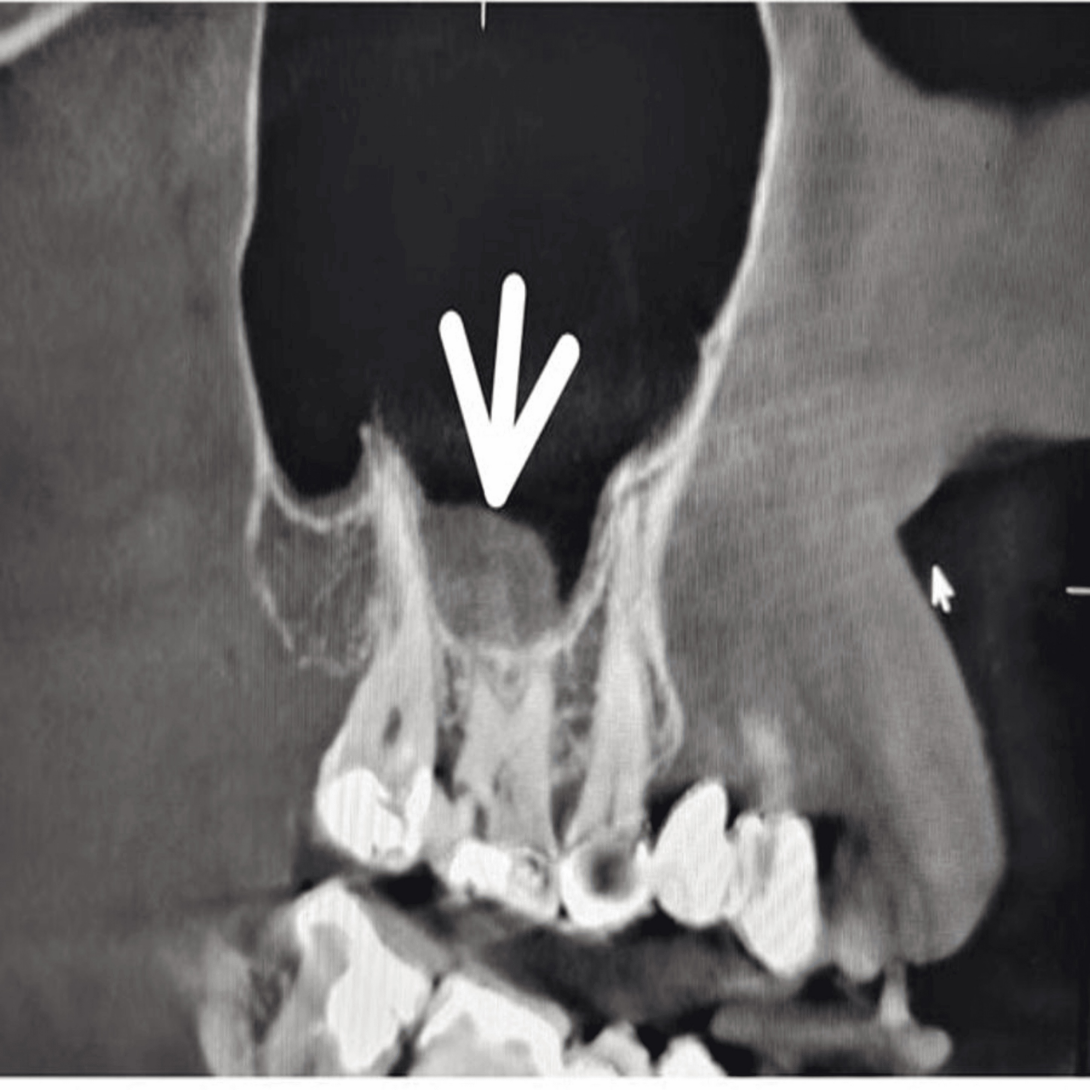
Observation of MSMT in the right MS in CBCT image. MSMT: Maxillary sinus mucosal thickening; CBCT: Cone beam computed tomography
